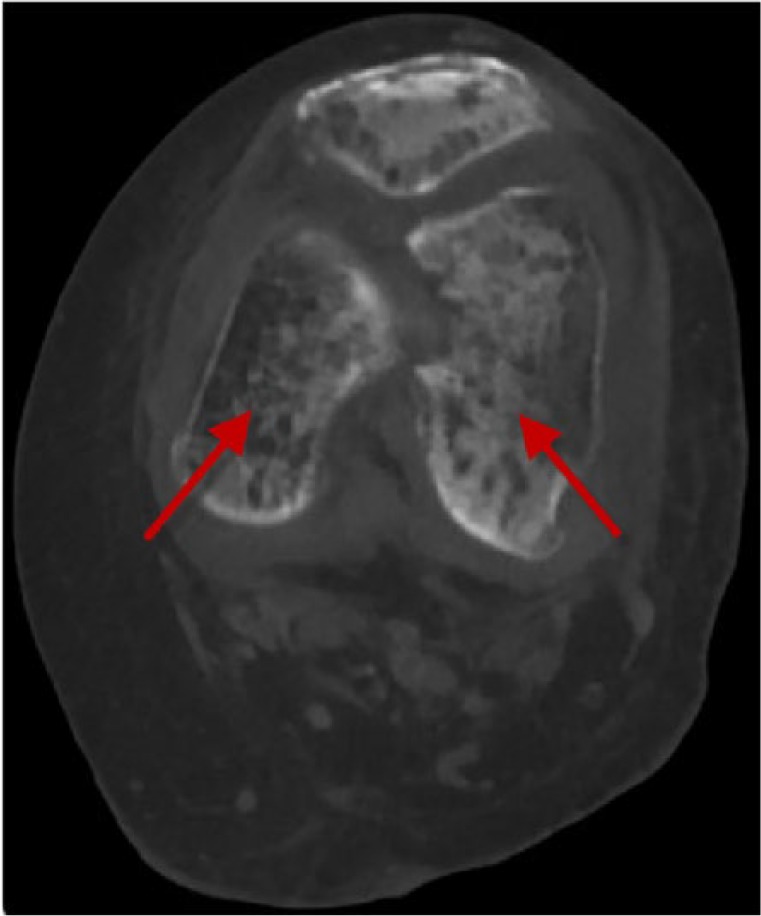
Enhanced computed tomography of the left knee showing acute pathological fracture of the left distal femur (arrows).
